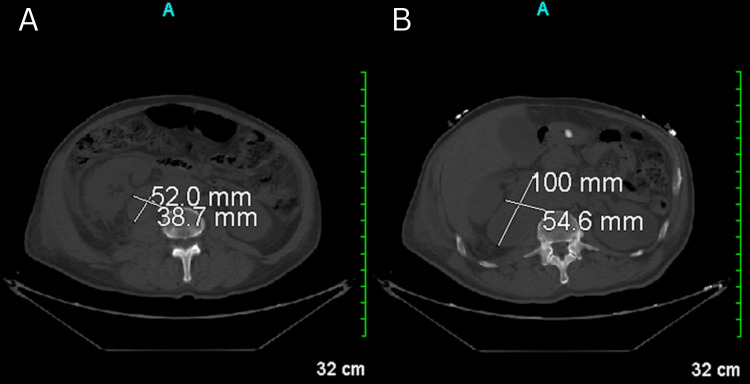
The CT scan reveals upper abdominal lymphadenopathy and extensive retroperitoneal lymphadenopathy. An index lymph node mass posterior to the right kidney and lateral to the inferior vena cava measures 5.2 x 3.9 cm (A), while another index lymph node mass in the upper abdomen measures 10 x 5.5 cm (B). Gallstones are present. Additionally, a small amount of ascites is observed. CT: computed tomography.
